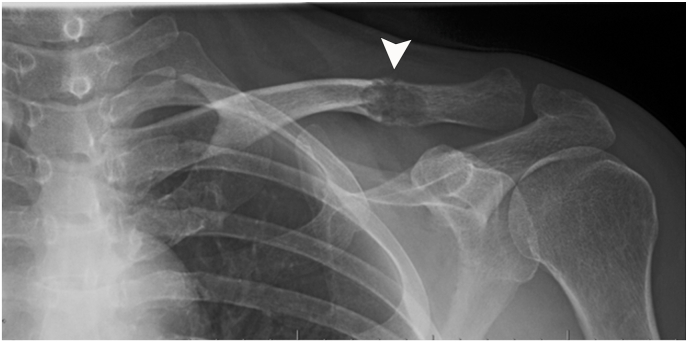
Radiograph of the left clavicle shows a lytic lesion (arrowhead) distally.
